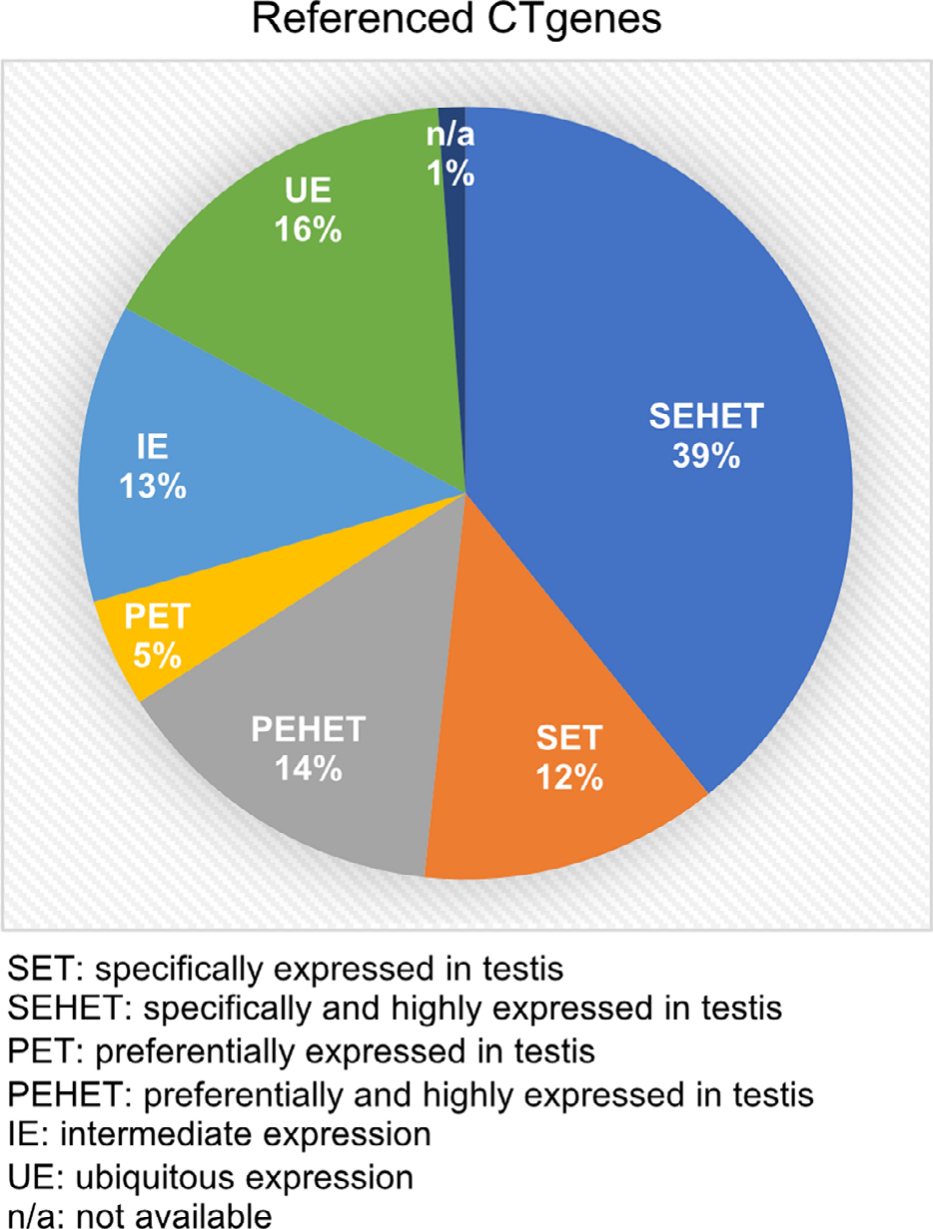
CT gene expression profiles. A pie chart shows the distribution of CT genes referenced in CT database (http://www.cta.lncc.br) among the expression classes defined by our analysis as indicated at the bottom. We employed probe sets for this analysis to avoid multiple allocations of genes to different classes. Percentages are rounded to the nearest integer.
